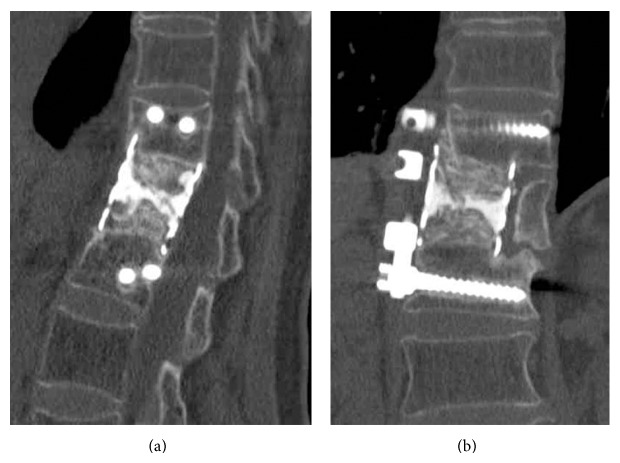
Computed tomography of the lumbar spine 2 years after surgery showing a bony fusion was successfully achieved without a recurrence of infection. (a) Sagittal view. (b) Coronal view.
